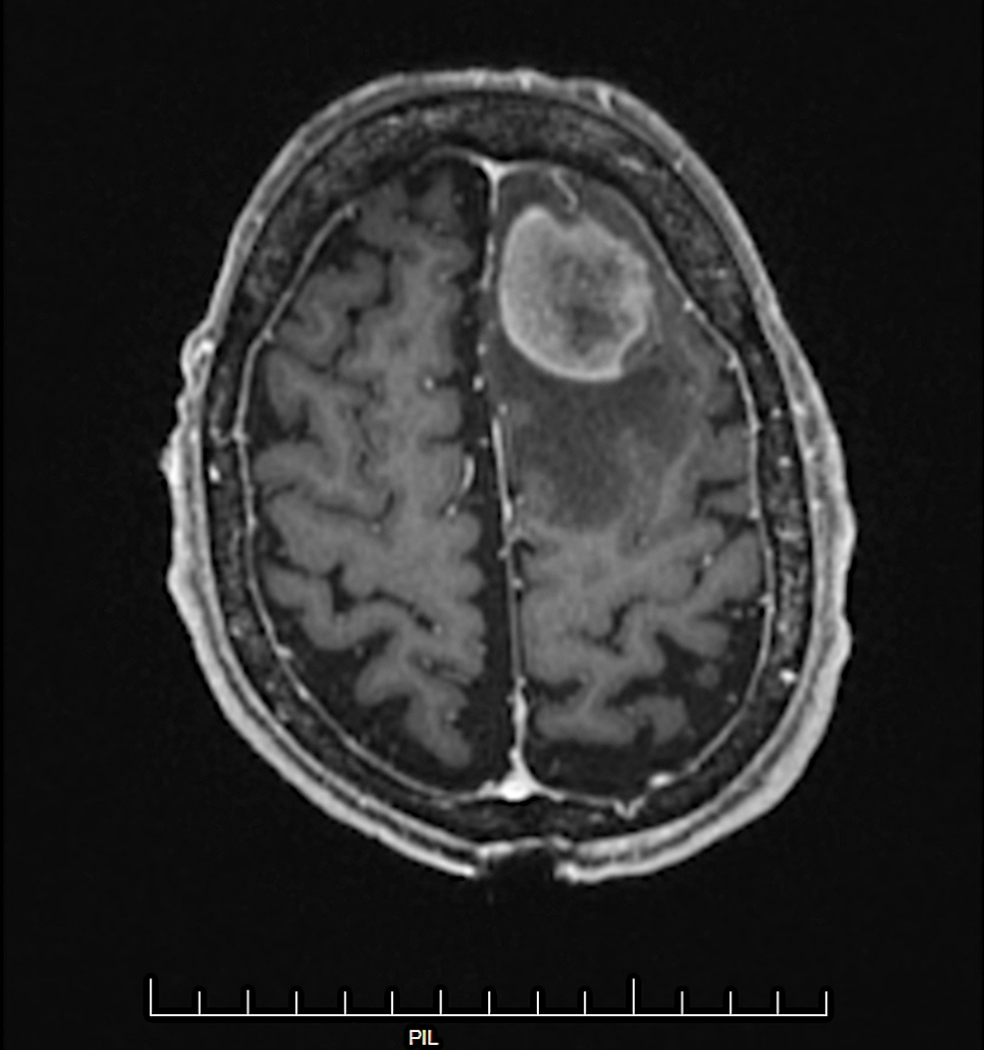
MRI brain displaying heterogeneous enhancement of a well-marginated 3.8 cm mass in the left frontal lobe anterosuperiorly with surrounding vasogenic edema.
